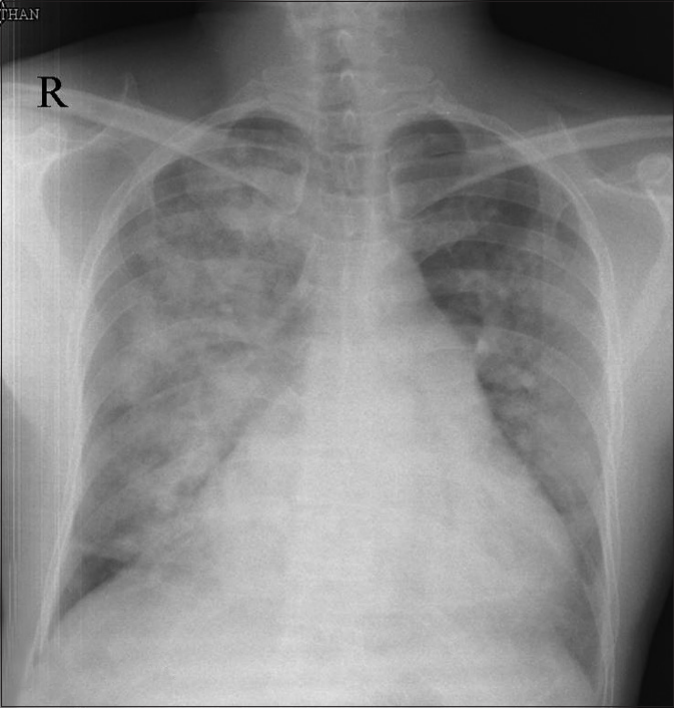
X-ray chest in postero-anterior view 18 hours after atrial septal defect device closure showing evolving pulmonary edema
